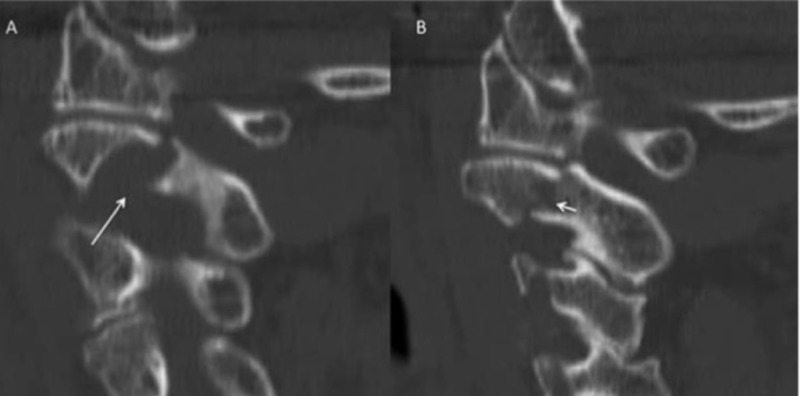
Sagittal noncontrasted CT scan. (A) Large vertebral foramen at C2-3 (long arrow) with a fracture superiorly in the C2 pars on the right side. (B) Displaced fracture through the left C2 pars and pedicle (short arrow).
